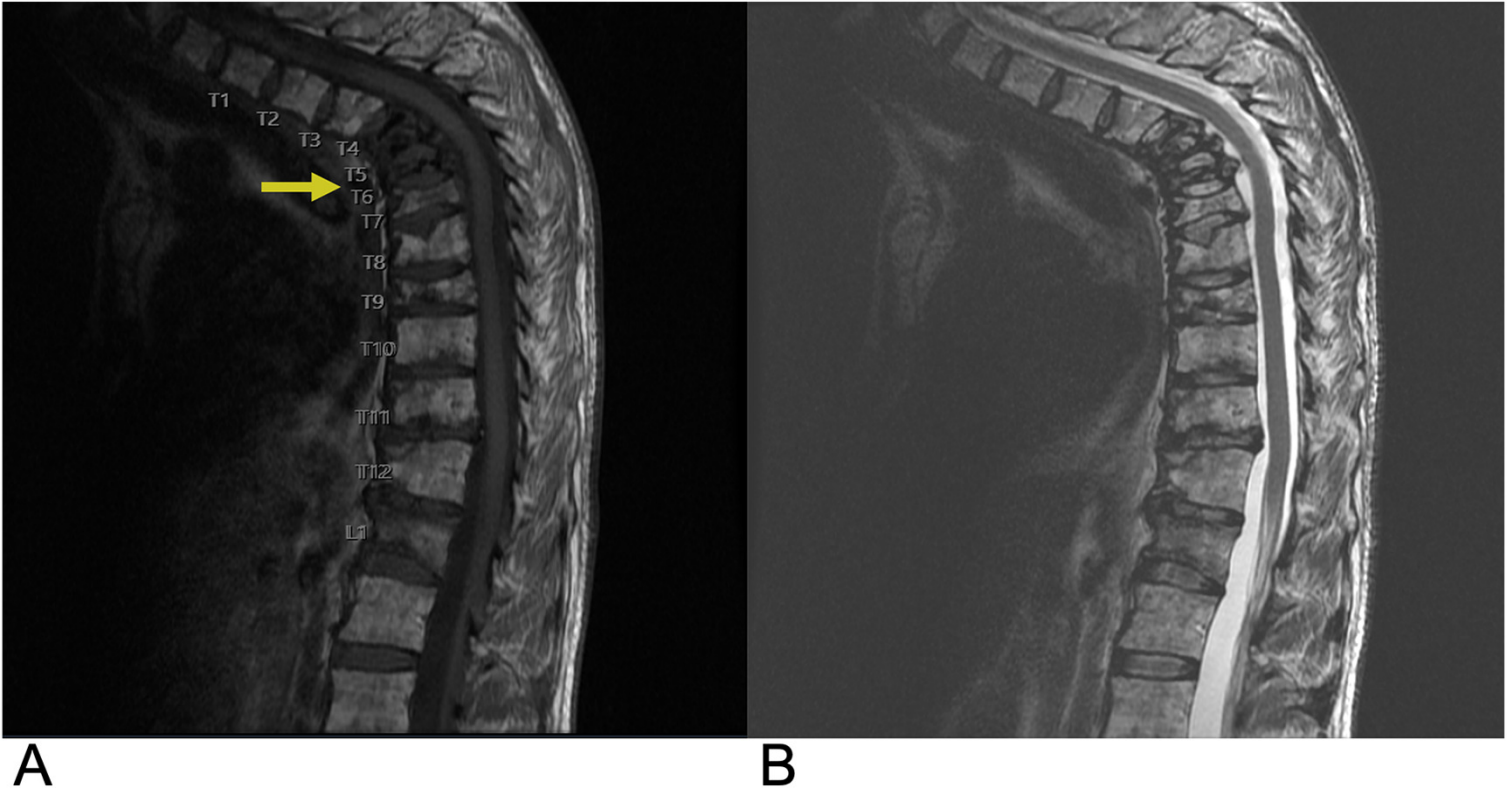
Vertebral compression fractures. Sagittal T1 (A) and sagittal STIR (B) MRI of the thoracic spine demonstrating osteoporotic compression fracture of upper thoracic vertebrae (yellow arrow) with height loss.
